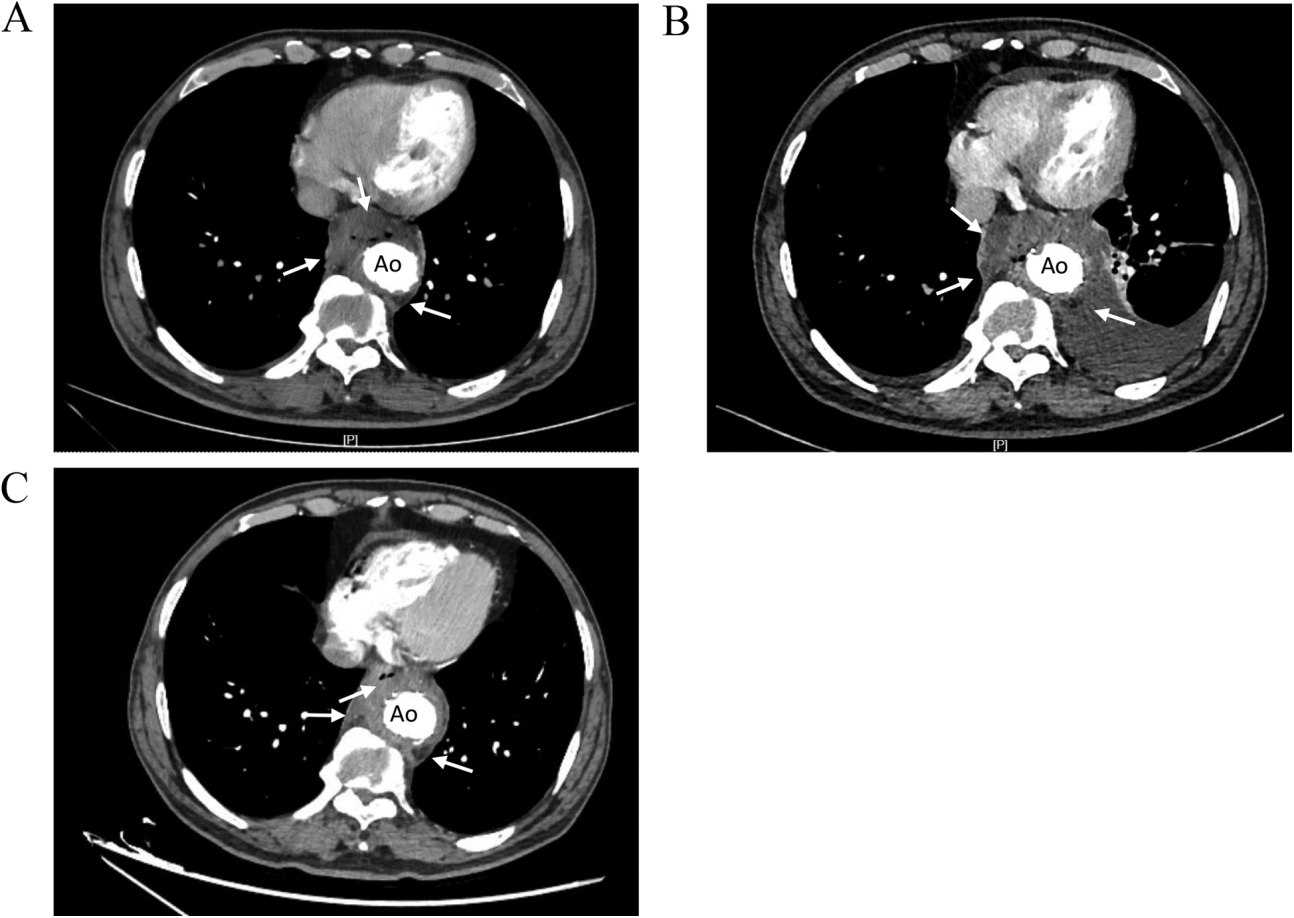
Comparison of thoracic aortic CTA images showing. (A) CTA images 2 months before admission. Low-density shadow was found around the stent in the lower thoracic aorta (right side, right anterior, left posterior), and the boundary with the esophagus was not clear. (B) CTA images during hospitalization. The low-density shadow around the stent in the lower thoracic aorta had unclear boundary with the esophagus, accompanied by air in it, and the scope of the lesion was larger than before. (C) CTA images at 3 months after discharge. The mass lesion around the lower thoracic aortic stent with air accumulation in it, the range of infection was smaller than before. Ao, descending thoracic aorta.
